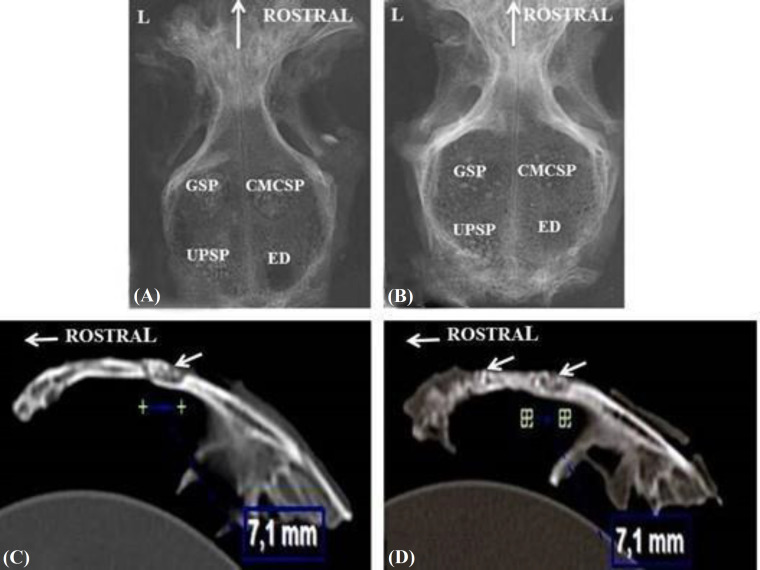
Radiographic views of the defects sites at the end of the (A) 1^st^ and (B) 3^rd^ months after the surgery. Vertical arrows indicate rostral direction. (C) A CT image of the UPSP-filled defect at the end of the 1^st^ month; horizontal arrow indicates rostral direction and the oblique arrow indicates the defect site. (D) A CT image of the GSP (the left oblique arrow) and CMCSP-filled (the right oblique arrow) defects at the end of the 3^rd^ month after the surgery; horizontal arrow indicates rostral direction. Symbols on Figures 2C and 2D show the areas measured
